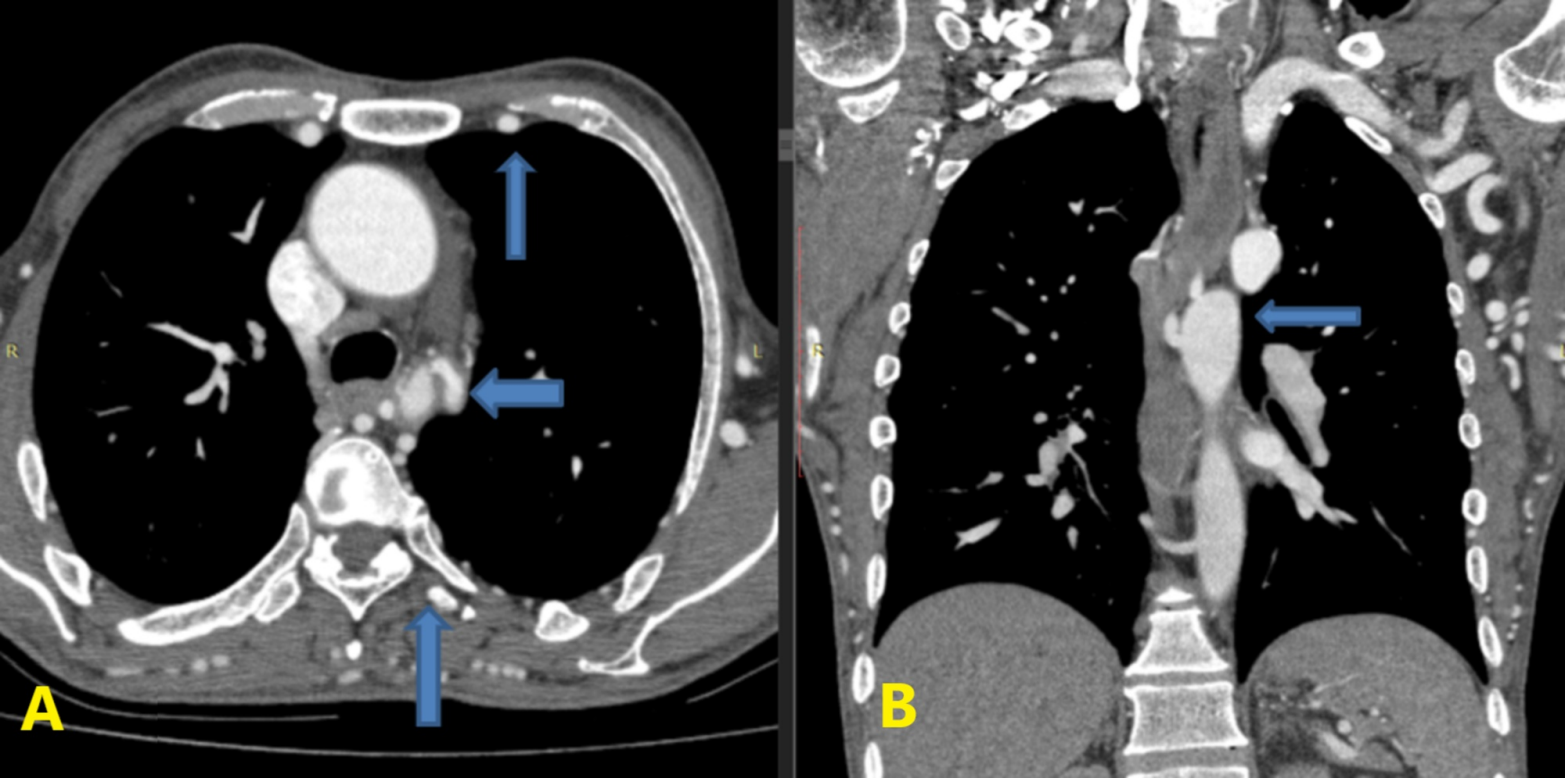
Axial CT scan showing a narrowed segment with enlarged internal thoracic and intercostal arteries (A) and coronal view demonstrating coarcted segment (B).
